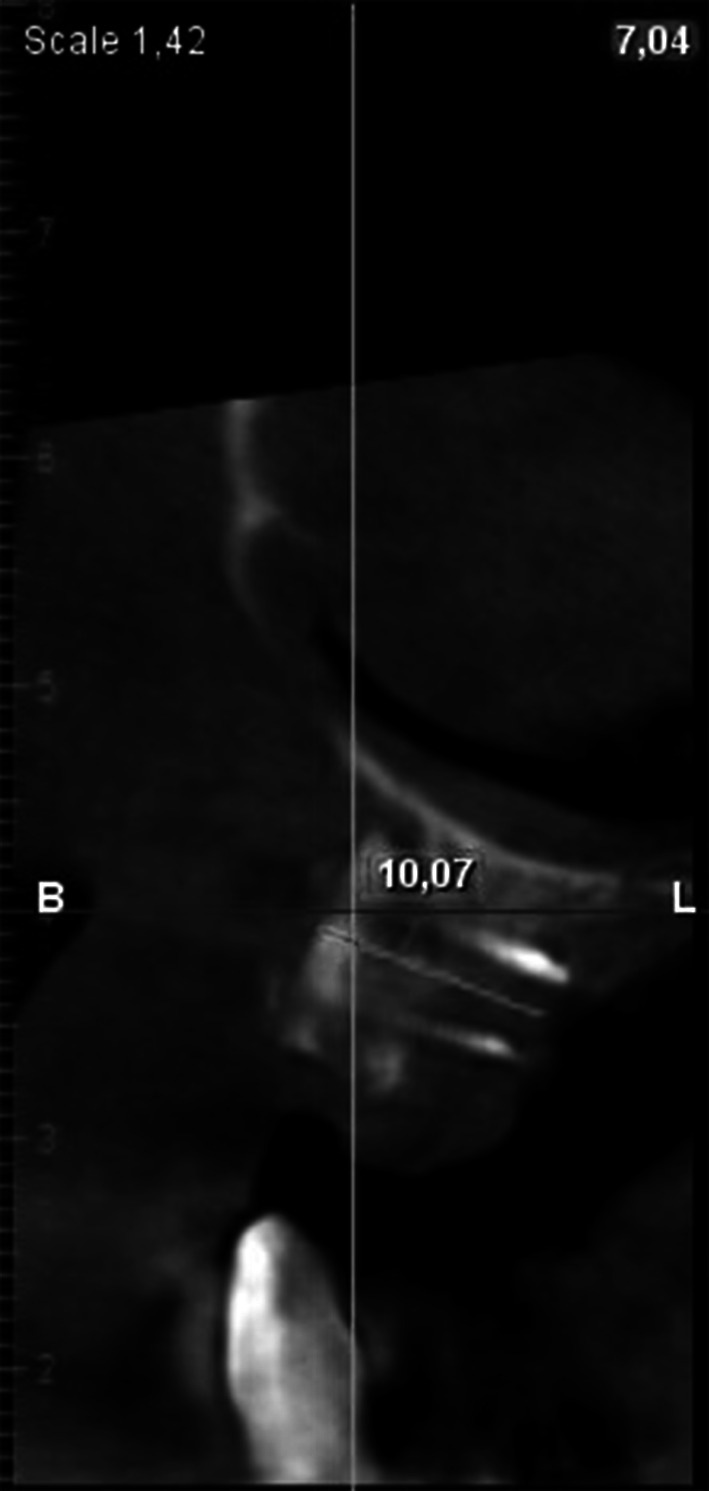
CT after grafting, the same location
